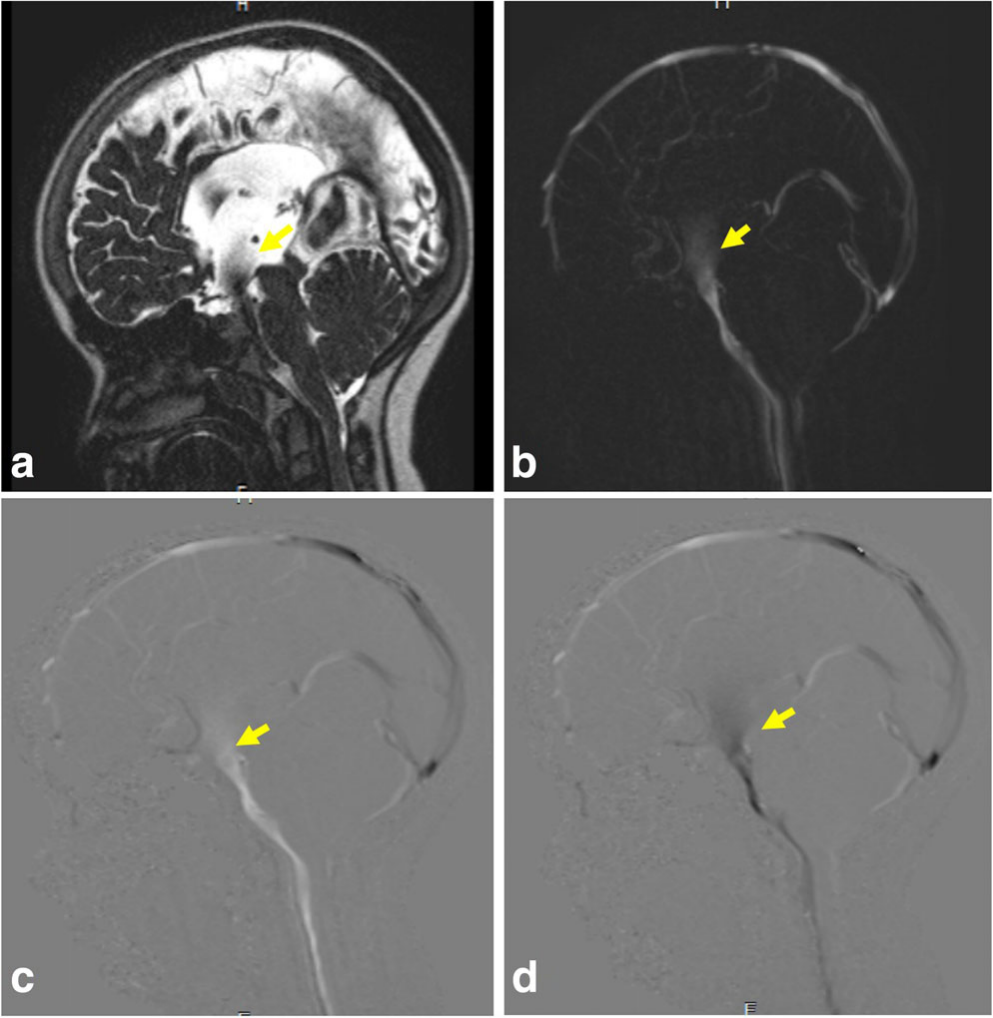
A 9-year-old girl with ventriculostomy. a 3D-DRIVE demonstrates flow void through a defect in the third ventricular floor (arrow). b Phase-contrast MRI reveals flow jet through the ventriculostomy (arrow). c, d Phase images through systole and diastole demonstrate the pulsatile flow throughout the cardiac cycle (arrow)
